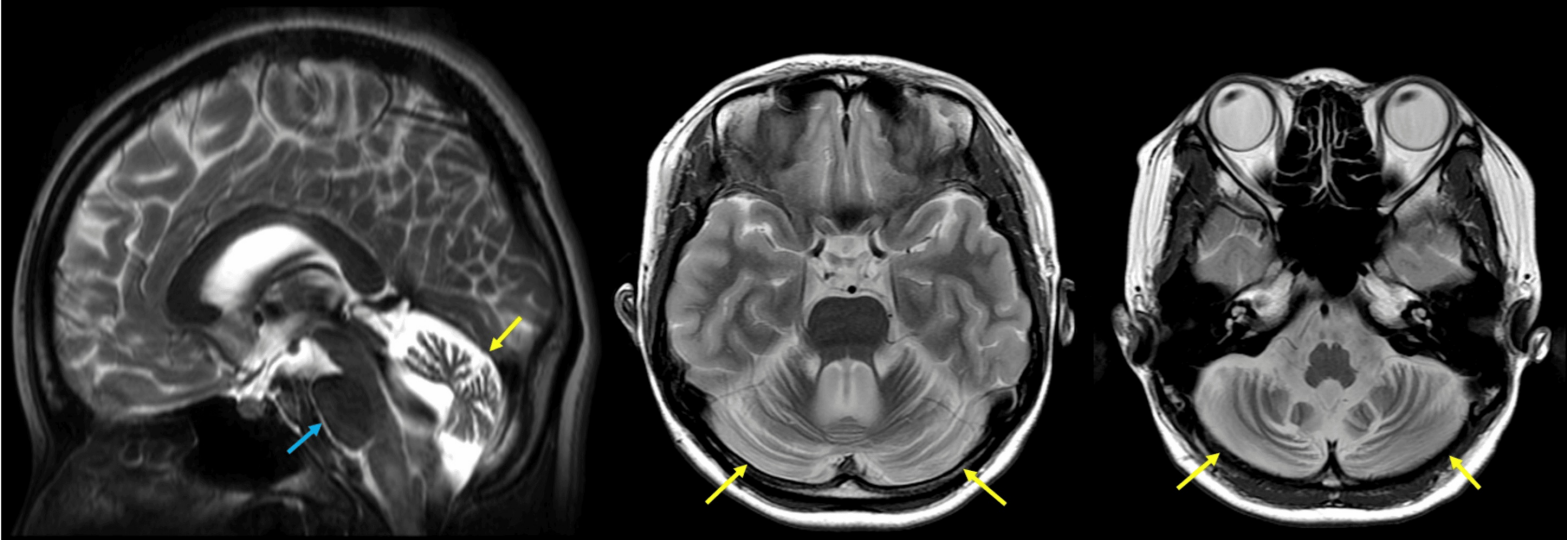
The result of brain magnetic resonance imaging (MRI) at 28 years old. T2-weighted brain MRI shows significant cerebellar atrophy (yellow arrows) and mild brainstem atrophy (blue arrow).
